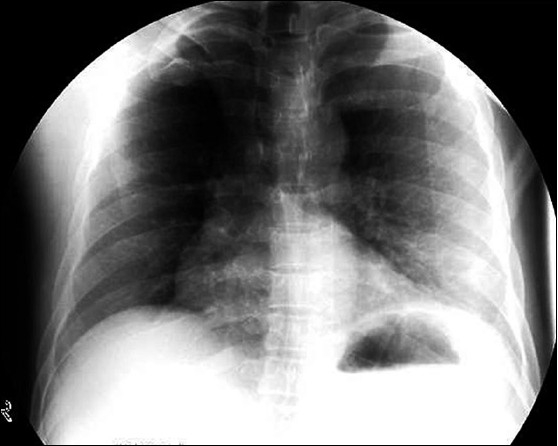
Chest radiograph showing an elevated left hemidiaphragm
